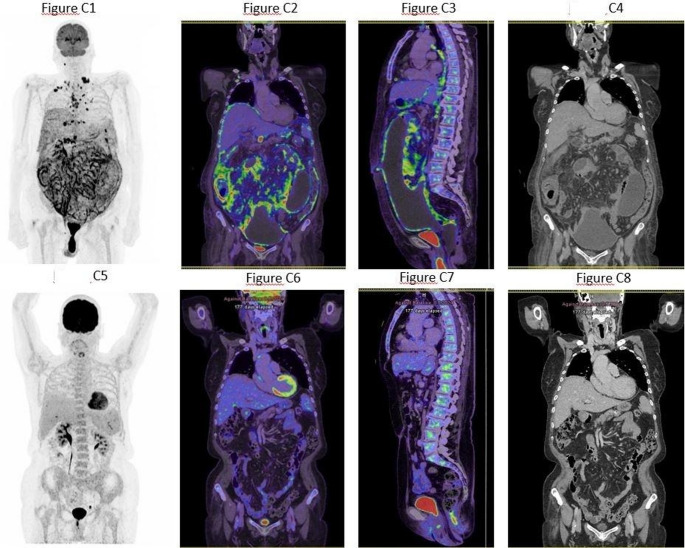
FDG-PET / contrast-enhanced CT Images at baseline (upper row) and after treatment (lower row). Maximum Intensity Projection (MIP) at baseline showing diffuse metabolic activity in lymph nodes of the neck, mediastinum, and epigastrium. Diffuse hypermetabolism is seen in the intra-abdominal cavity along the mesentery and peritoneum (C1). After treatment, complete resolution of supradiaphragmatic lymph nodes is observed. A new active focus in the liver parenchyma and low-grade persistent metabolic activity on the caudal surface of the liver are noted, while other peritoneal and mesenteric foci have disappeared (C5). Diffuse mesenteric and peritoneal wall hypermetabolic changes and a good treatment response are visualized in coronal and sagittal fusion images (C2, C3, C6, C7). Contrast-enhanced CT shows nodulation, diffuse mesenteric edema, and ascites, as well as their resolution after treatment (C4, C8)
